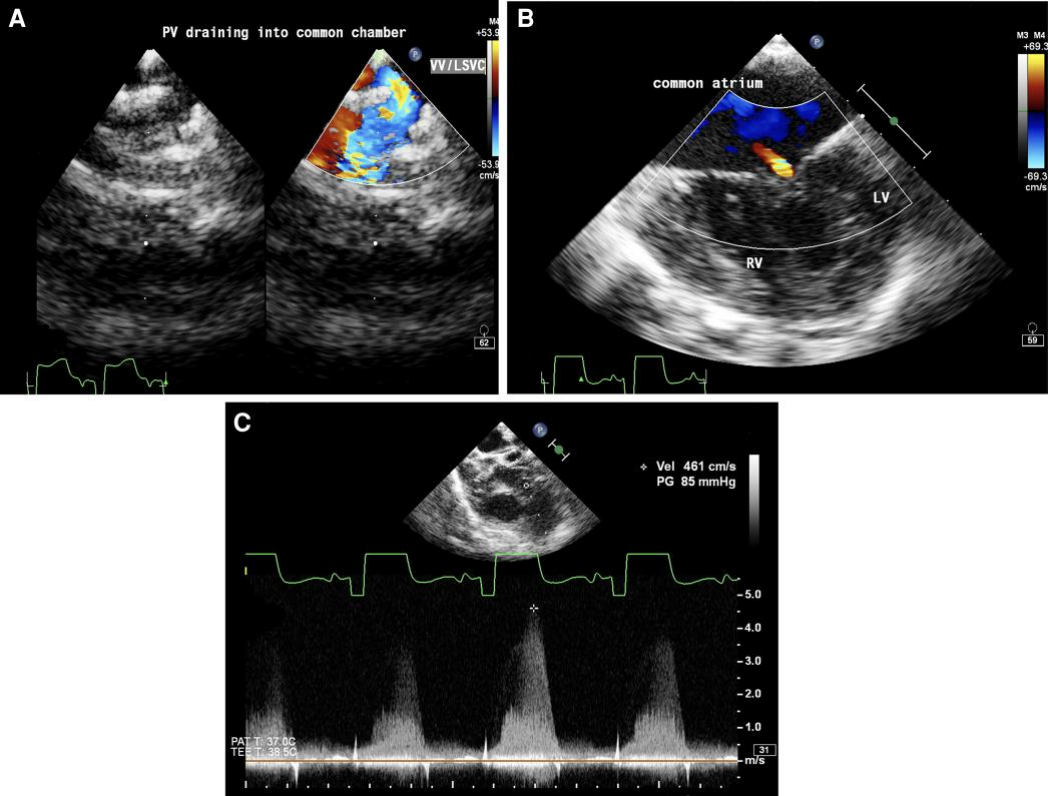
Transoesophageal echocardiogram (*A*) upper oesophageal view with colour Doppler showing pulmonary veins draining into vertical vein (VV)/left superior vena cava (LSVC). (*B*) Mid-oesophageal four-chamber view showing a common atrium, hypoplastic left ventricle (LV), well-formed right ventricle (RV), and mild common atrioventricular valve regurgitation. (*C*) Aortic short axis view with continuous wave Doppler across pulmonary valve showing pulmonary stenosis with a gradient of 85 mmHg.
